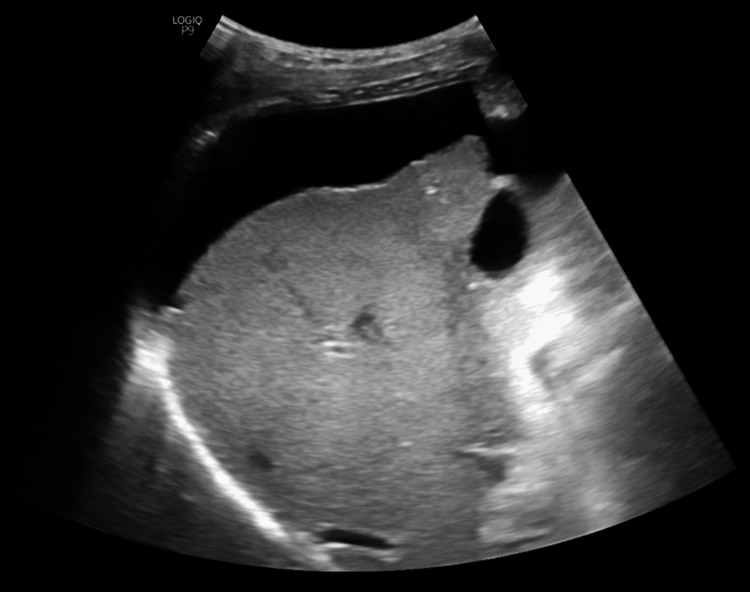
USG abdomen of case 3. Ultrasound (USG) image reveals a coarsened echotexture of the liver with an irregular surface and ascites.
